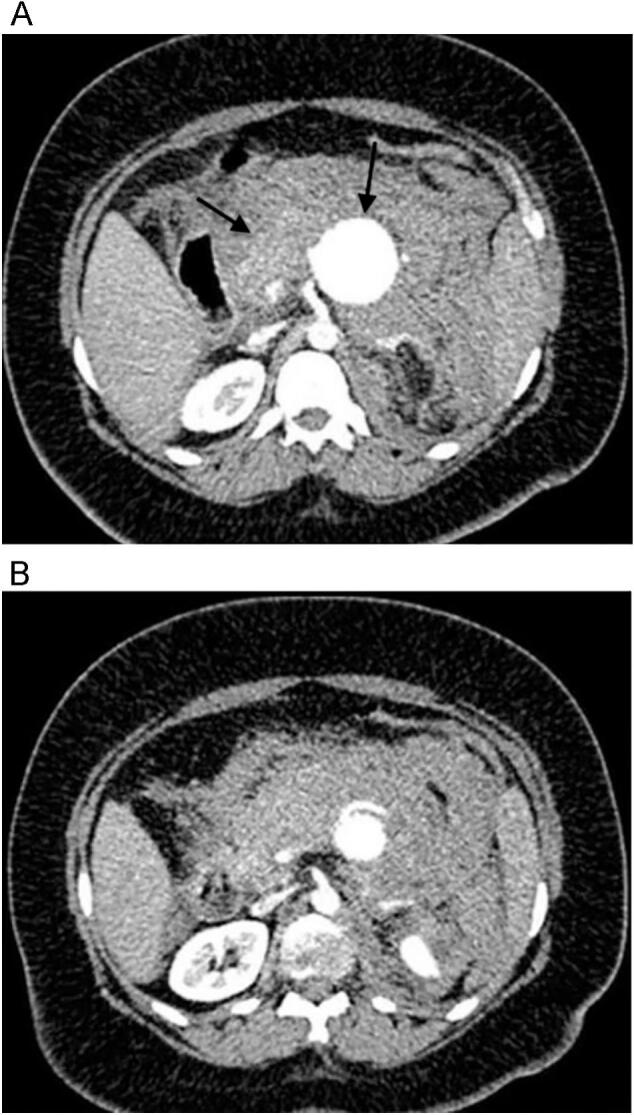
Pretreatment contrast-enhanced CT is showing an aneurysmatic dilation in the proximal of the splenic artery. Arrows: Aneurysm sac and retroperitoneal hematoma.
